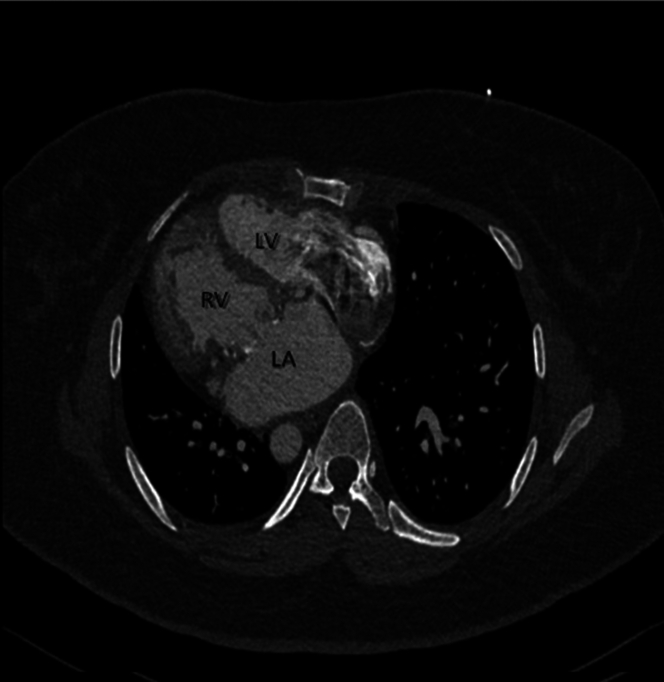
Computed Tomography Scan The scan demonstrates the unique cardiac anatomy of the patient at the level of the tricuspid valve. LA = left atrium; LV = left ventricle; RV = right ventricle.
